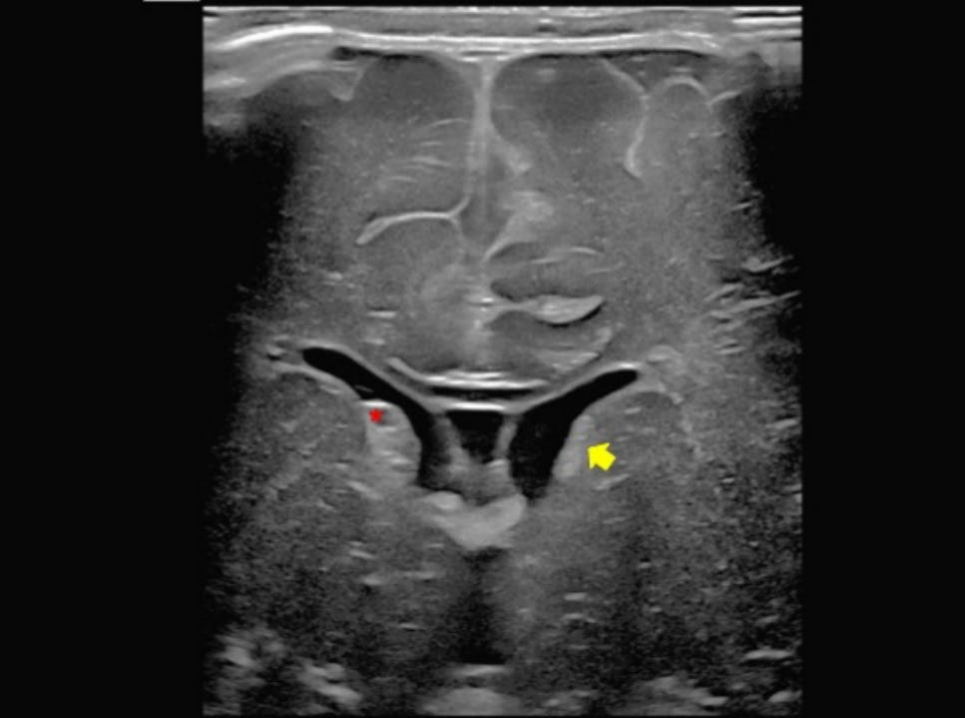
Premature male infant born at 34 weeks’ gestation who underwent cerebral ultrasound at 2-month old for fever (up to 38.7 °C) and anemia. Coronal plane well showed bilateral hemorrhage in the groove between the thalamus and the nucleus caudate. Grade 1 on the left side and grade 2 on the right side (yellow arrow and red asterisk, respectively)
